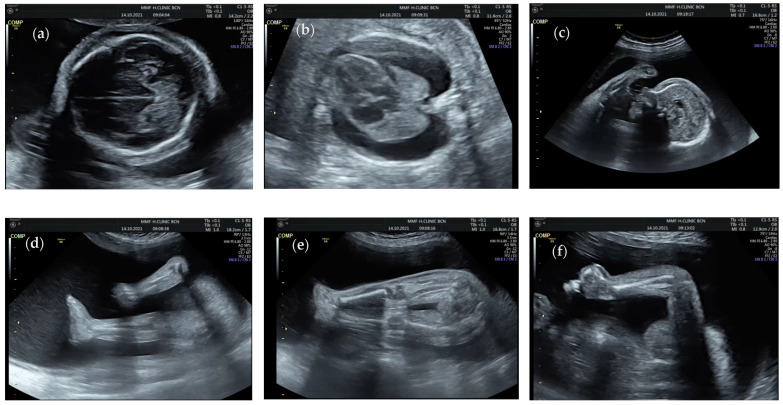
Transabdominal ultrasound images of the present case. Transabdominal ultrasound images of the present case showing (a) scalp edema, (b) subcutaneous edema and hydrothorax, (c) forehead edema, (d,e) lower extremity hyperextension, (f) upper extremity and hand contracture.
